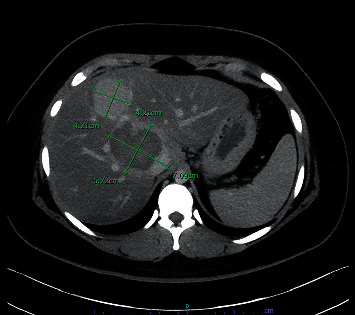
CT scan of the abdomen repeated in two months showing that the dominant HA decreased in size, measuring 7.1 × 5.7 cm, with coils and a resolved hemorrhagic component. The nonhemorrhagic mass in segment four decreased to 4.2 cm in diameter.
